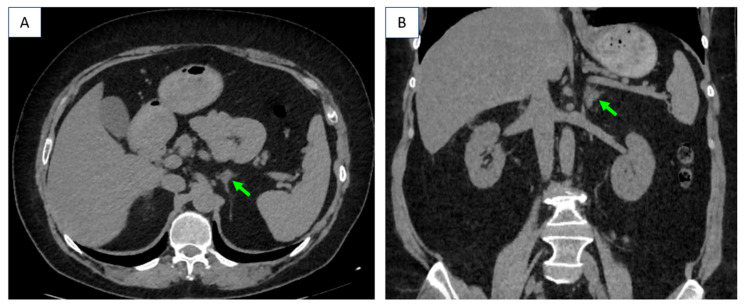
Six months after unilateral adrenalectomy, CT showed stationary left adrenal tumor (of 1.2 by 1.5 cm) [CT scan native phase highlights the tumor (green arrow): (A) axial plane; (B) coronal plane].
